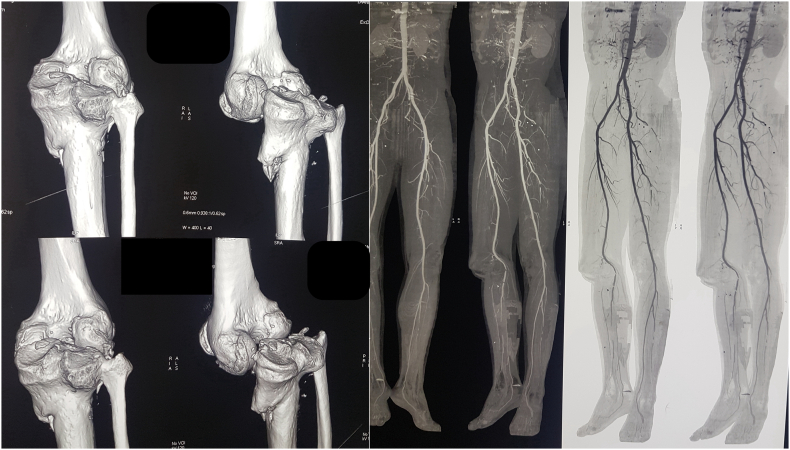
Computed tomography angiography showing normal arterial blood flow.
